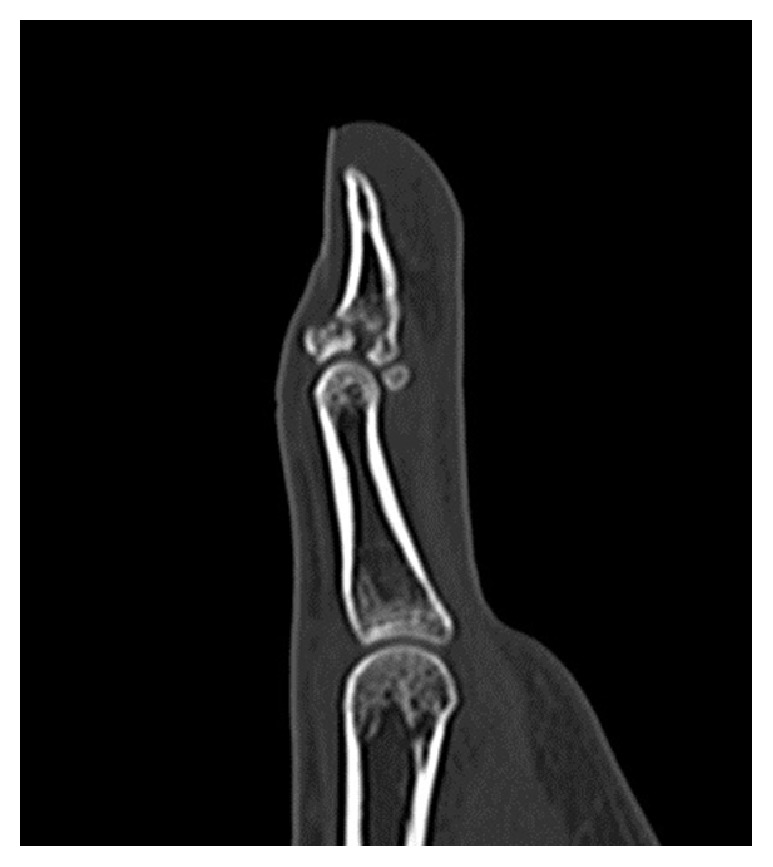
Computed tomography shows an avulsion fracture at the base of the distal phalanx involving more than half of the articular surface.
